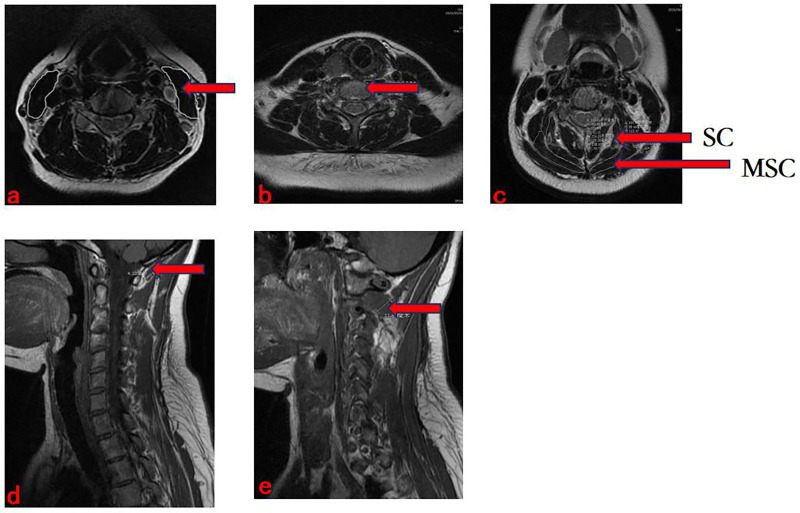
Muscle localization via neck MRI in a patient. (a) SCM(mid-level of C5 vertebral body); (b) LC(mid-level of C6 vertebral body); (c) MSC and SC(mid-level of C4 vertebral body); (d) RCPmi(lateral to the intervertebral joint, sagittal plane); (e) OCI(paraspinal, sagittal plane).
